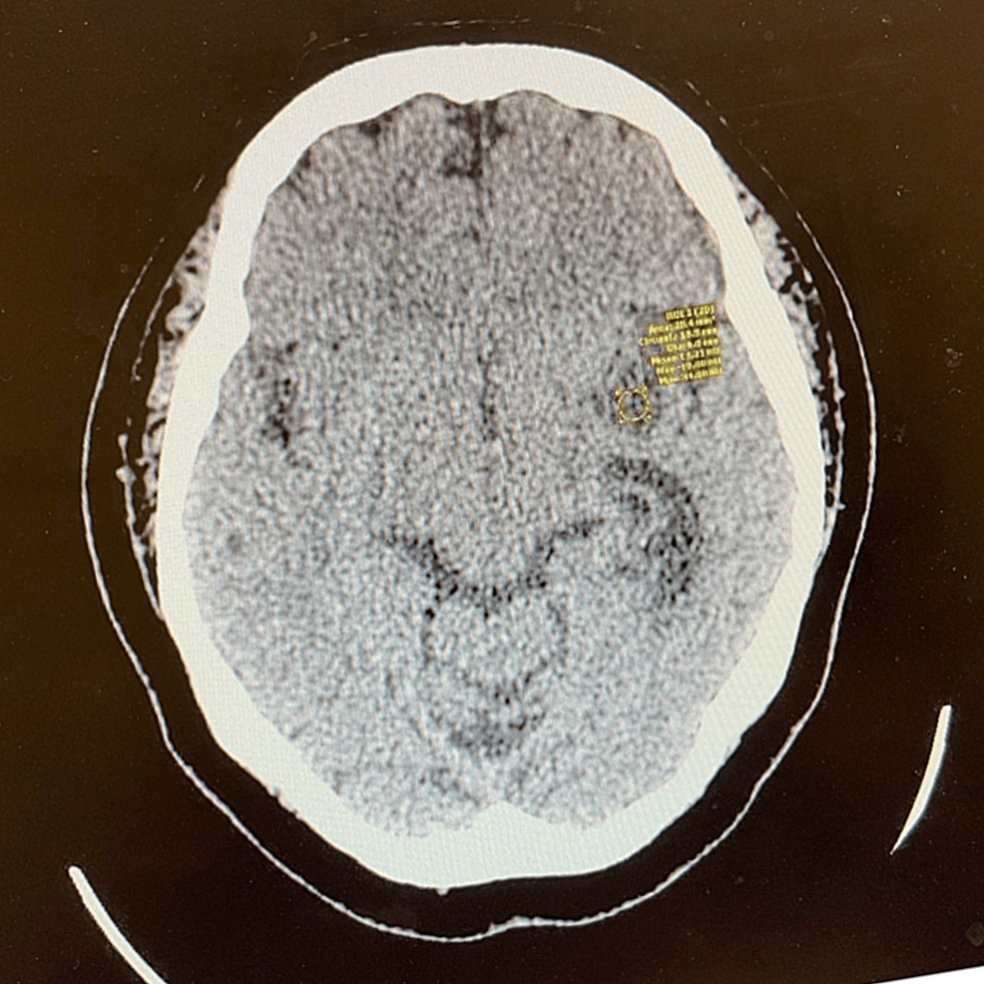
Computed tomography image showing the lesion in axial view.
